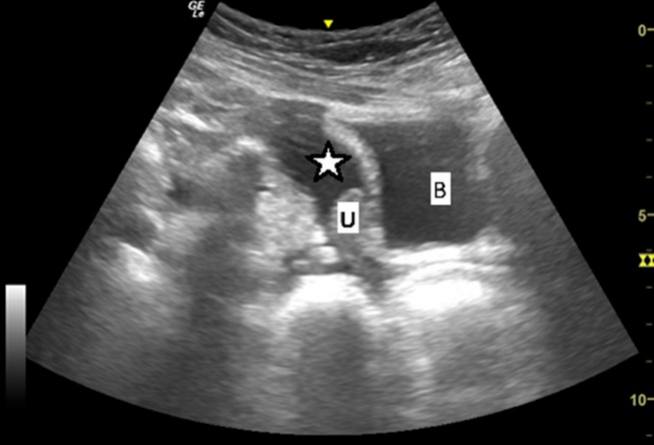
Point of care ultrasound (POCUS) image of pelvis in longitudinal plane using the curvilinear 5-12 MHz probe. This demonstrates a moderate amount of free fluid (*) in the pelvis. The bladder (B) is located inferior to this, and the uterus (U) is shown.
